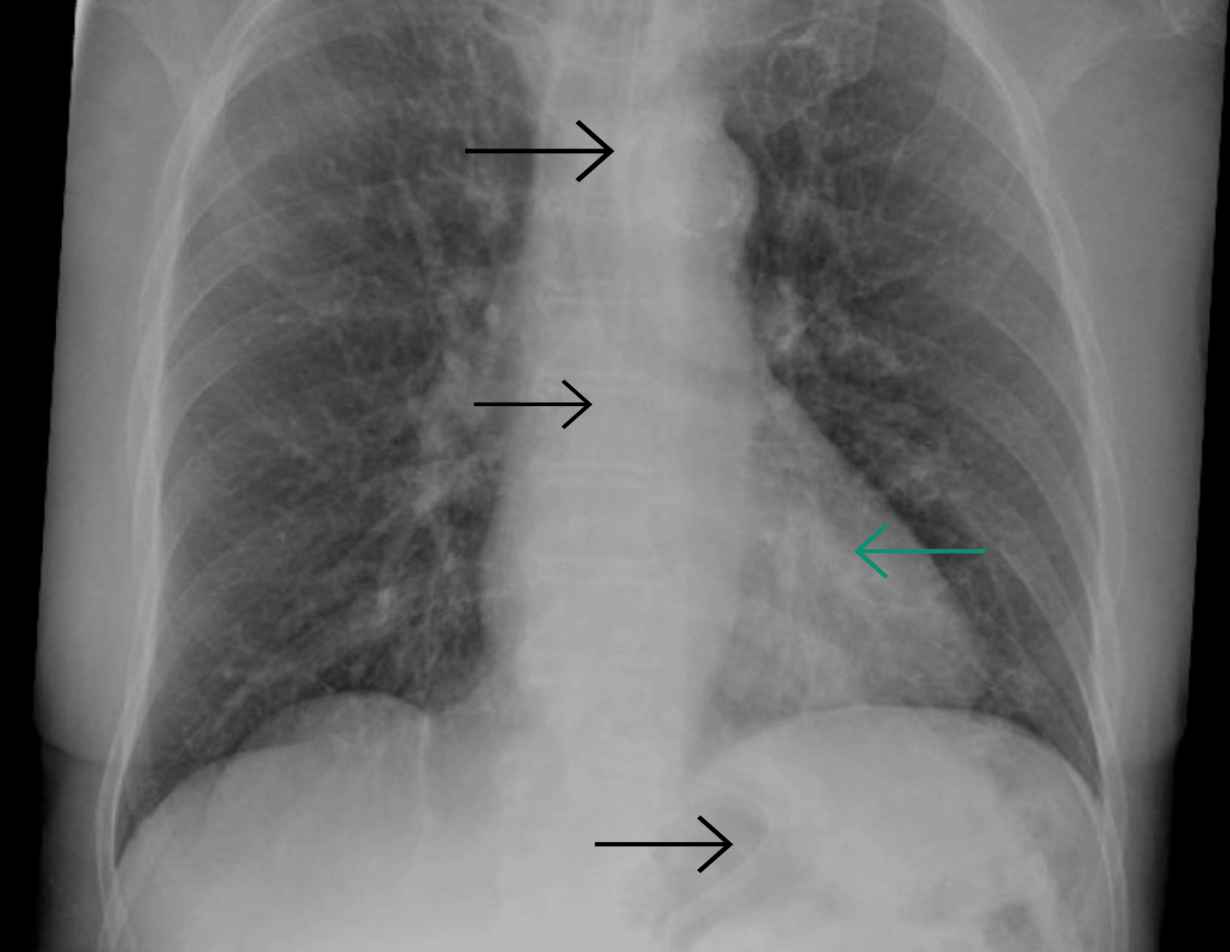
Chest X-ray showing suspected infiltrates in the left lower lung zone, in the retrocardiac location, with clear costophrenic angles. Black arrows indicate the nasogastric tube.
